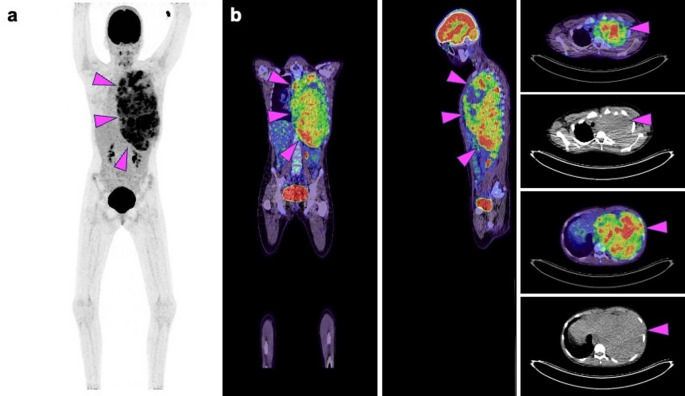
[^18^F]FDG PET/CT scan of an 11-year-old girl with a primary pleural extra-skeletal Ewing sarcoma (EwS). a: Maximum intensity projection (MIP) and b: exemplary transversal, sagittal and coronal slices of [^18^F]FDG PET/CT fusion (or corresponding low-dose CT) showing the mass with inhomogeneous, mostly intense [^18^F]FDG uptake (SUVmax: 11.3 and SUVmean: 5.5). The giant mass, measuring approximately 15 × 15 × 30 cm, occupied the entire left hemithorax without rib/bone involvement, and displacing the mediastinum to the right (magenta arrows)
